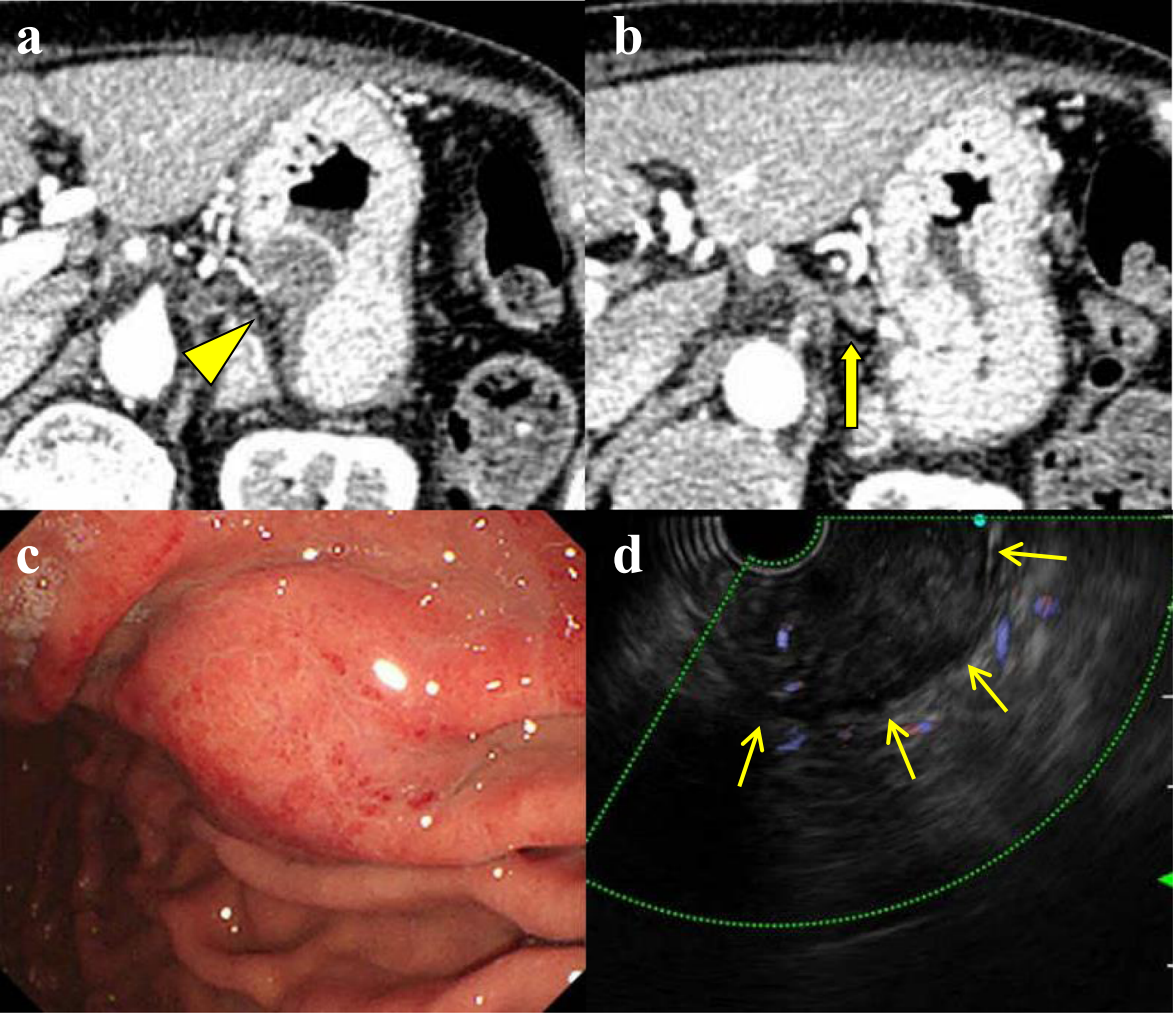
Images at the detection of needle tract seeding in the gastric wall. a Twenty-five months after initial pancreatectomy, computed tomography revealed a 23-mm-diameter low-density mass in the wall of the gastric body (arrow head). b A lymph node around the stomach was swollen (arrow). c Gastroendoscopy revealed an approximately 10-mm-diameter submucosal tumor in the posterior gastric wall. d Endoscopic ultrasound revealed a hypoechoic mass in the submucosa (arrows)
